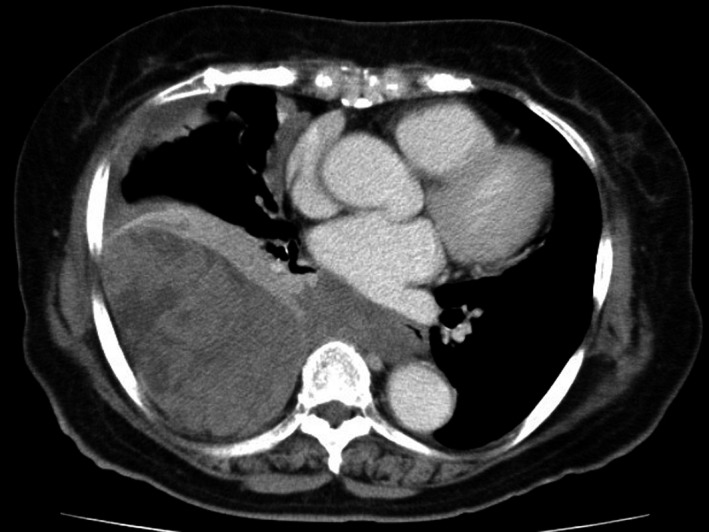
Computed tomography showed a large, well‐circumscribed mass with pleural effusions.
